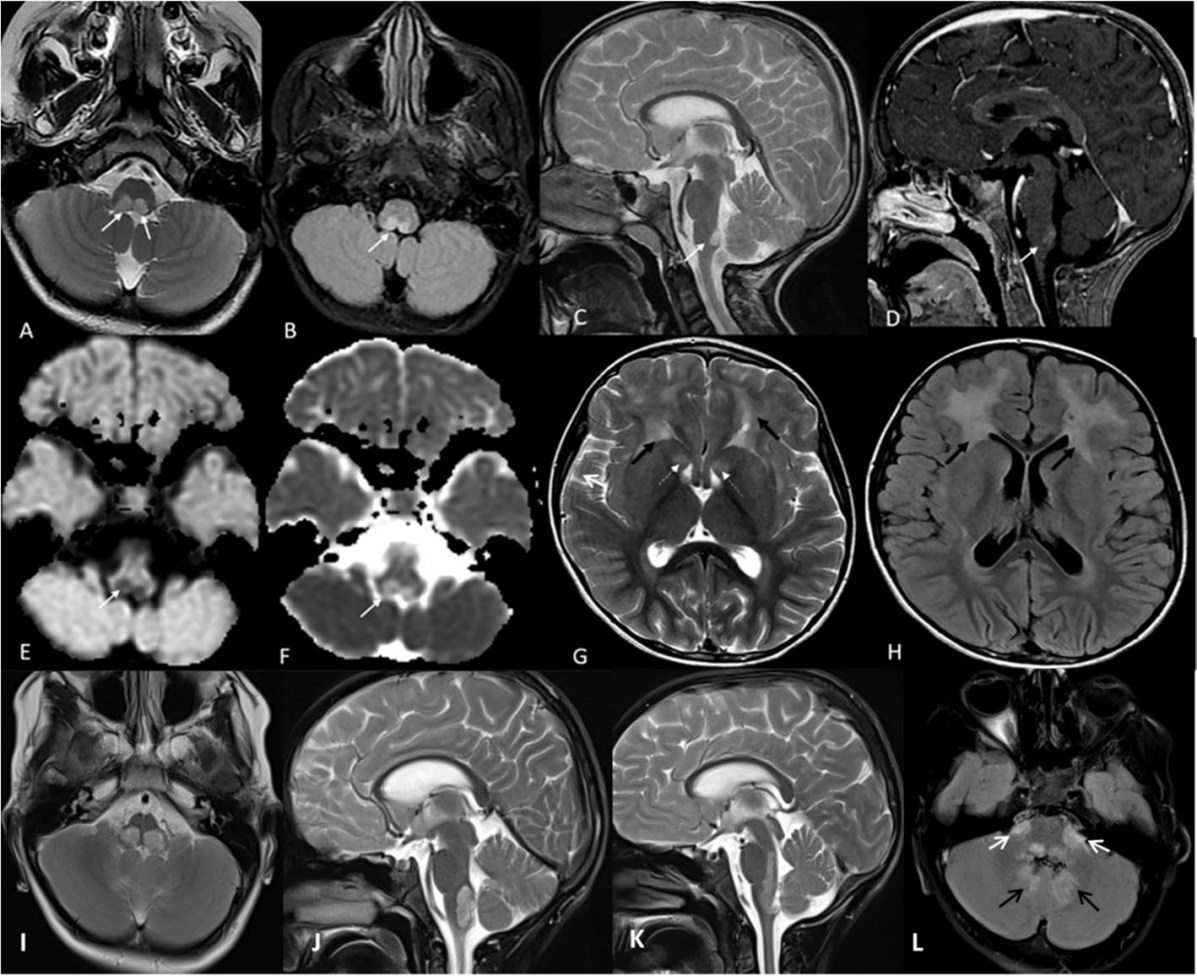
The patient’s brain magnetic resonance imaging (MRI) findings. MRI data demonstrated bilateral lesions (white arrows) in the posterior aspect of medulla oblongata near the floor of the fourth ventricle ( known as area postrema), which was hyperintense on T2-W (A and C) and FLAIR (B) sequences with some enhancements on T1-W post gadolinium administration sequence (D) and with no restricted diffusion on DWI (E) and ADC map (F) sequences. The involvement of periventricular white matter (especially in frontal lobes) with extension to subcortical white matter containing few small cysts (black arrows in G and H) and hyperintensity of basal ganglia in caudate heads (white dashed arrows in G). Third-row images belong to follow-up MRI at the age of 8 years including axial and sagittal T2-W (I, J, K) and Axial FLAIR (L); enlargement of the lesion in area postrema (I and J) extending to posterior pons (K) and involvement of bilateral middle cerebellar peduncles and dentate nuclei (white and black arrows in L, respectively)
